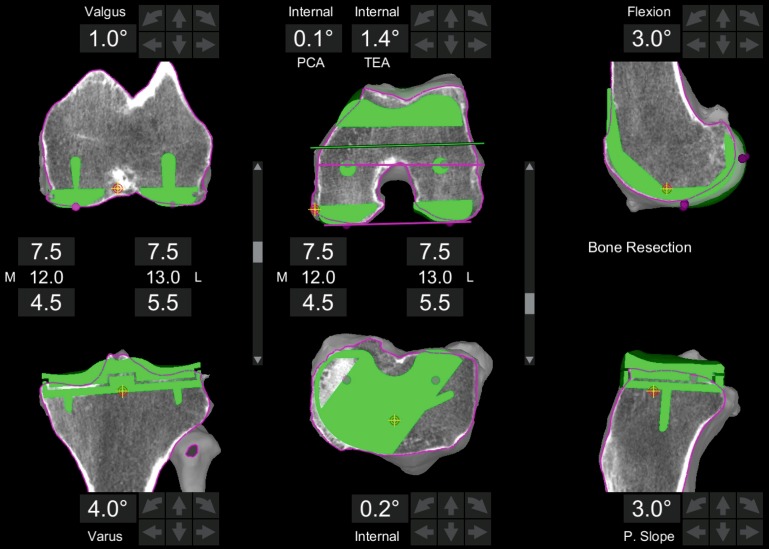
Screenshot of a prosthetic preplanning based on a patient individual knee model from the CT scan. *Left* is the frontal, in the *middle* the axial and on the *right* the sagital CT view with the prosthesis displayed in *green*. Prosthetic alignment is based on the principle of kinematic alignment with symmetric 7.5 mm resection on the distal and dorsal femur. The tibia is aligned to the surface of the proximal jointline. The resulting component orientation is outputted in degrees to the mechanical axes
